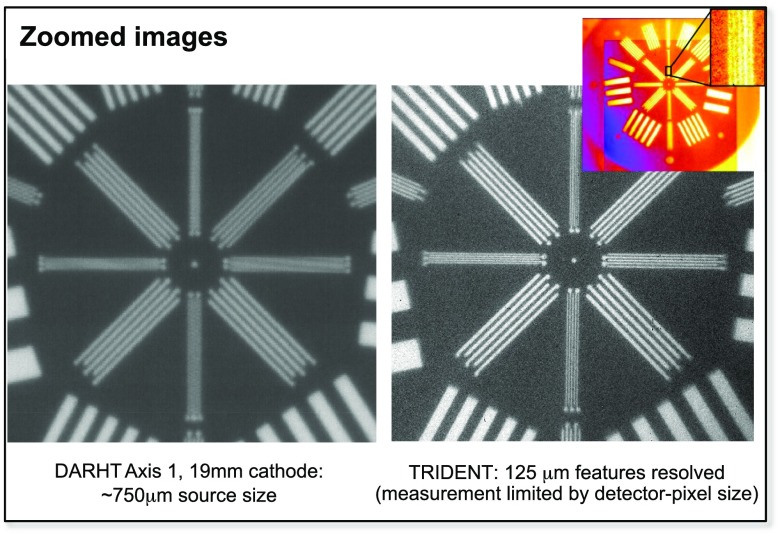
Gamma-ray point-projection radiographs of the AWE Kaleidoscope object, taken at DARHT (left) and at Trident (right).
